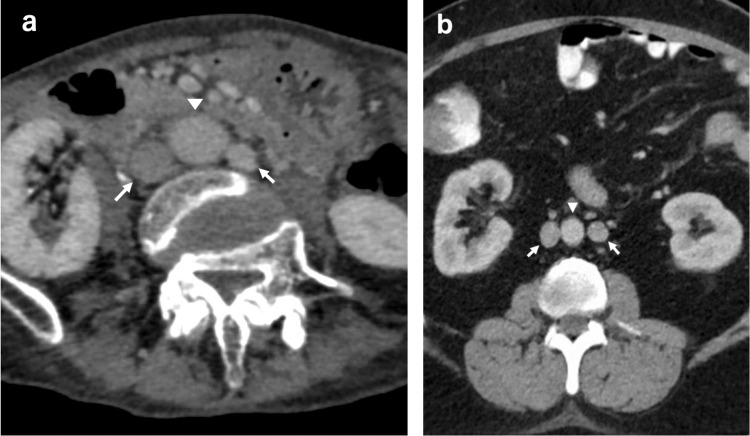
Axial CT images IVC: inferior vena cava (a) Our case shows duplicated inferior vena cava (IVC), with arrows indicating the right- and left-sided IVC, and the triangle indicating the abdominal aorta. (b) Reference image reproduced from Li et al. [1], showing a similar pattern of duplicated IVC
